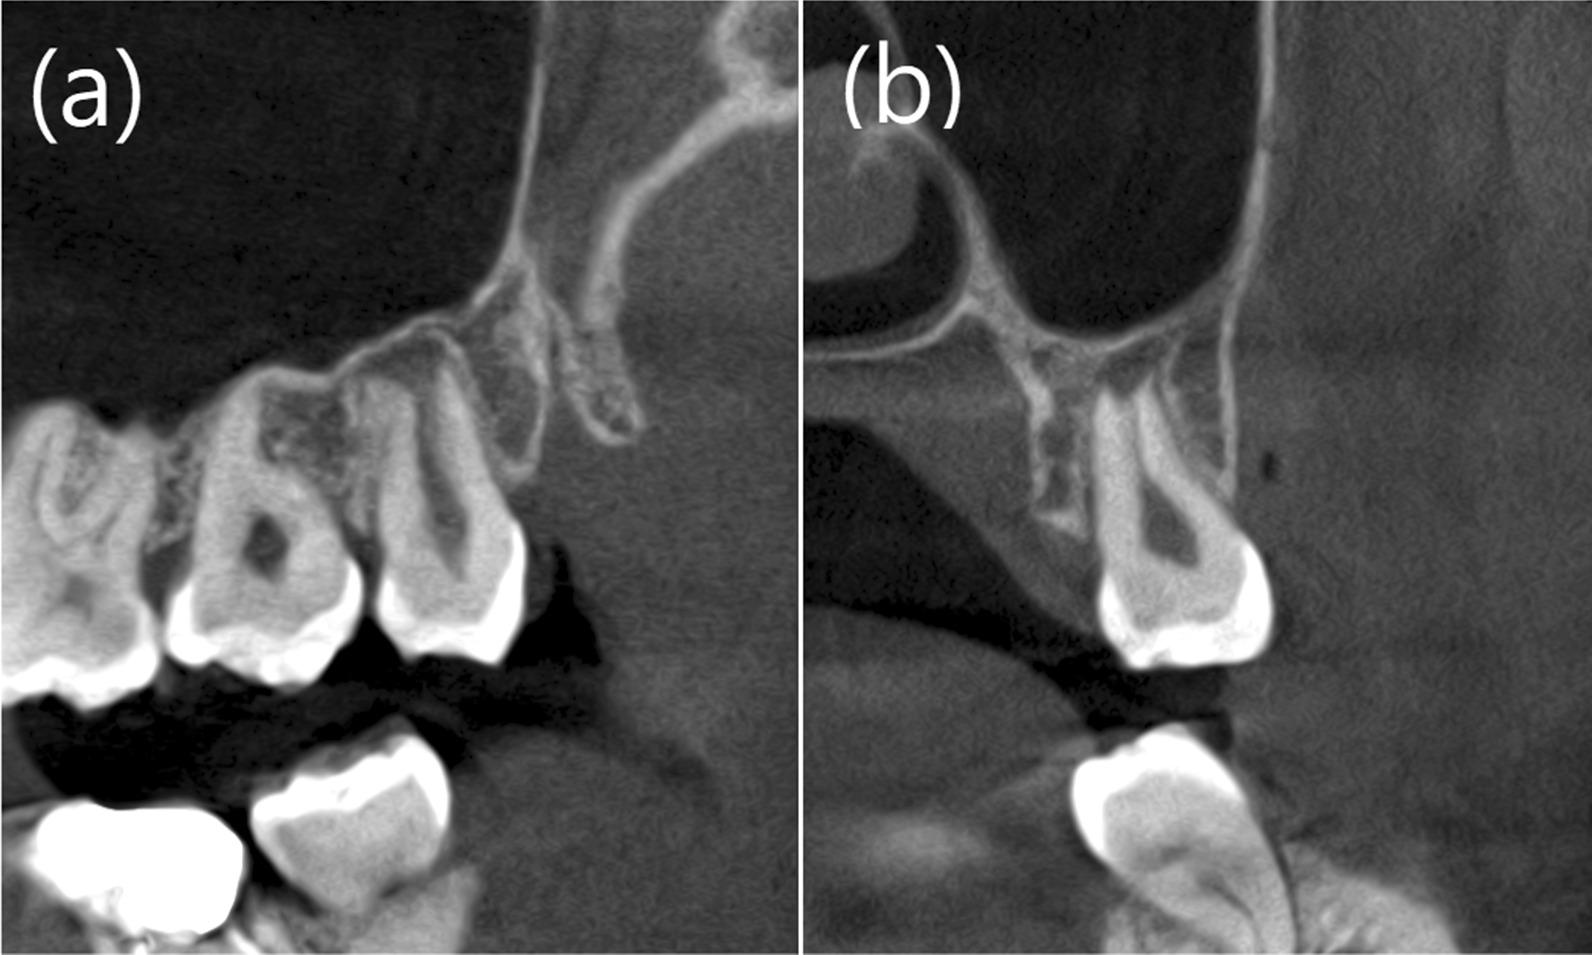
The Cone-Beam CT of example patient (Patient 2). a Sagittal view of the donor tooth 28. b Coronal view of the donor tooth 28
